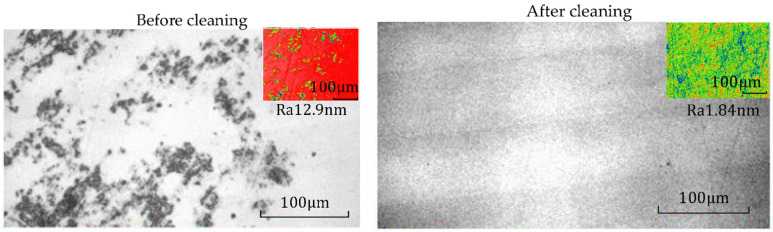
Surface roughness of a modified layer before and after ultrasonic cleaning.
